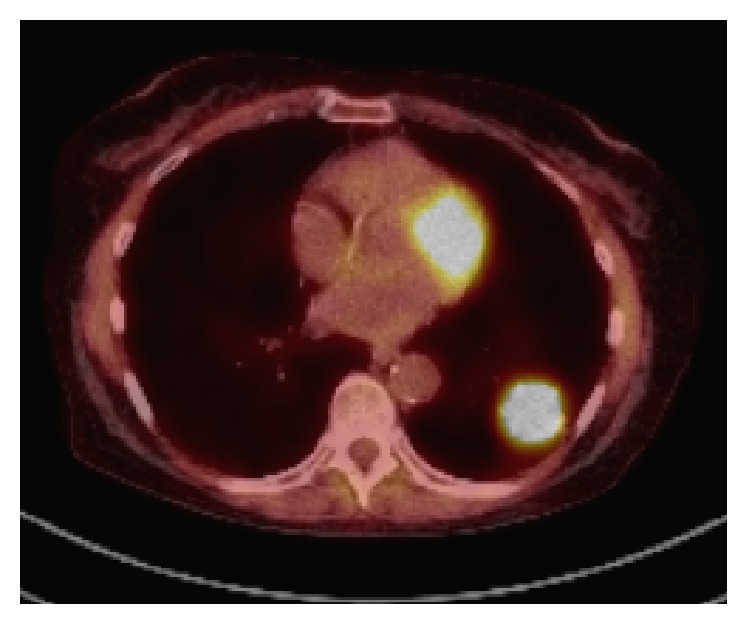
Fused PET/CT axial image shows the left lung mass.
